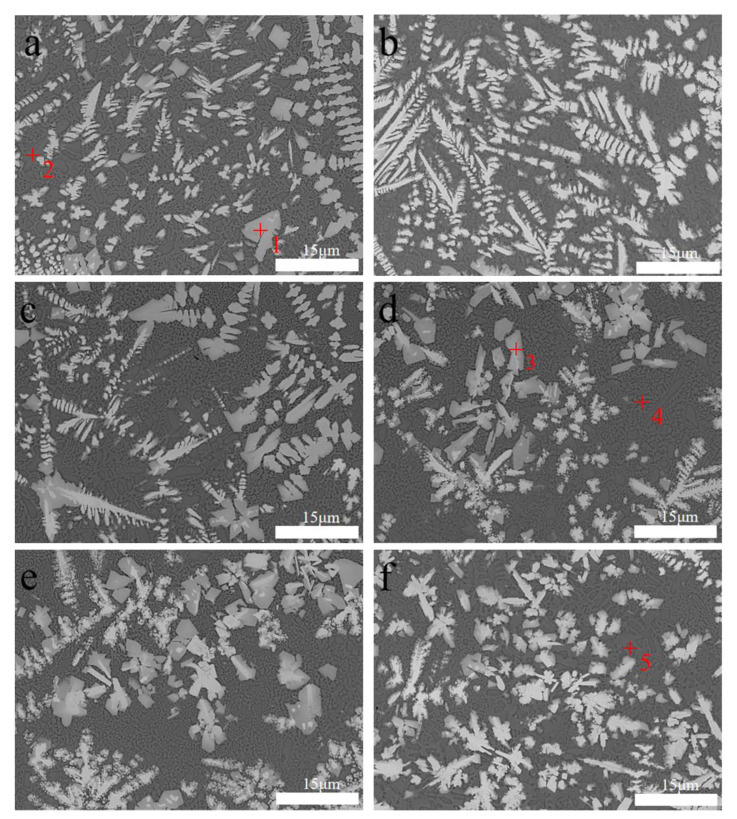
Microstructure of the middle part of the cladding layer subjected to different ultrasonic frequencies: (a) 0 kHz, (b) 22 kHz, (c) 27 kHz, (d) 32 kHz, (e) 37 kHz, (f) 42 kHz.
